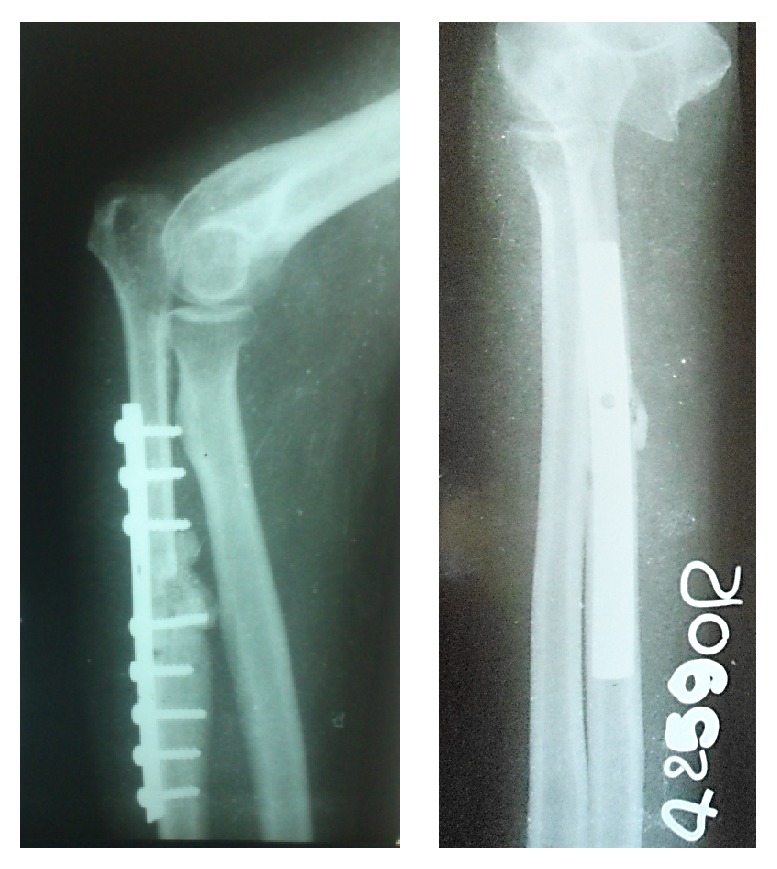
Follow-up X-ray after 2 years.
